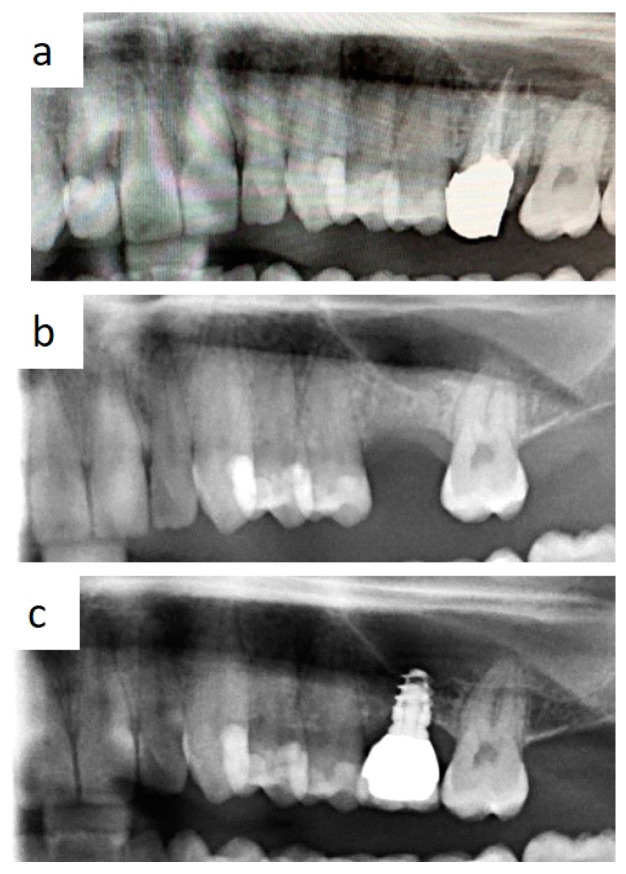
Occurrence of severe atrophy after removal of decayed first molar with periapical lesions (a,b); single tooth prosthetic rehabilitation without conventional sinus lift is feasible with short implant use (c).
